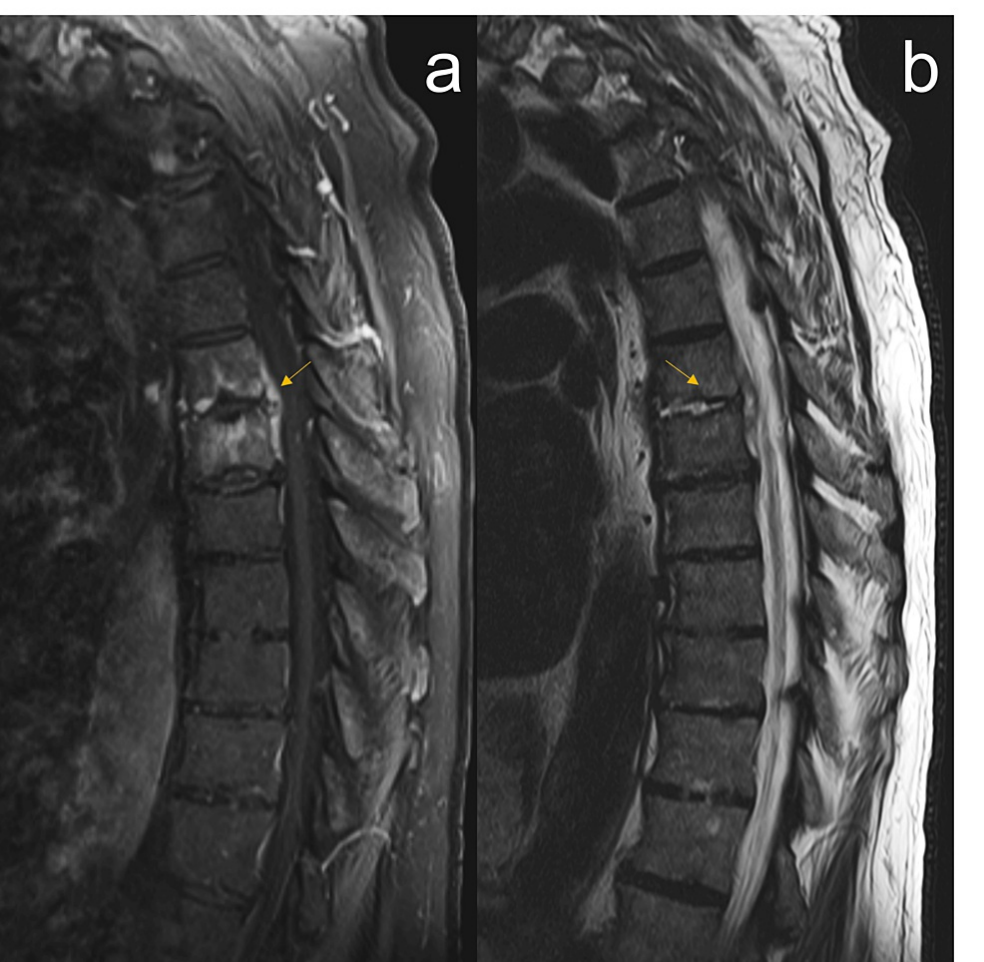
MRI thoracic spine with and without contrast, sagittal view. (a) T1-weighted FS demonstrating increased paraspinal soft tissue enhancement extending into the anterior epidural space with a six-millimeter collection along the left anterior epidural space concerning for a small epidural abscess at T6-T7 (arrow). (b) T2-weighted image demonstrating increased signal at T6-T7 disc space (arrow) associated with bone marrow edema and increased bone marrow enhancement compatible with acute discitis and osteomyelitis. MRI: magnetic resonance imaging; FS: fat saturation.
